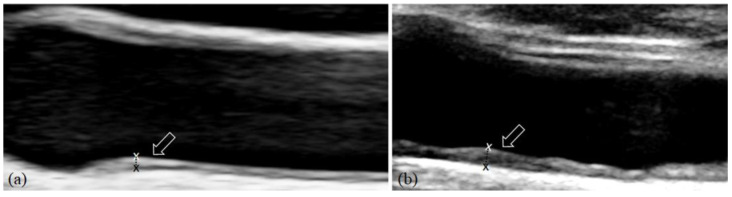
Longitudinal ultrasound of the common carotid artery. Intima-media thickness (IMT) was measured (callipers and arrowed) at the far wall of the distal portion of the common carotid arteries close to the carotid bulb. (a) Normal and (b) thickened IMT.
